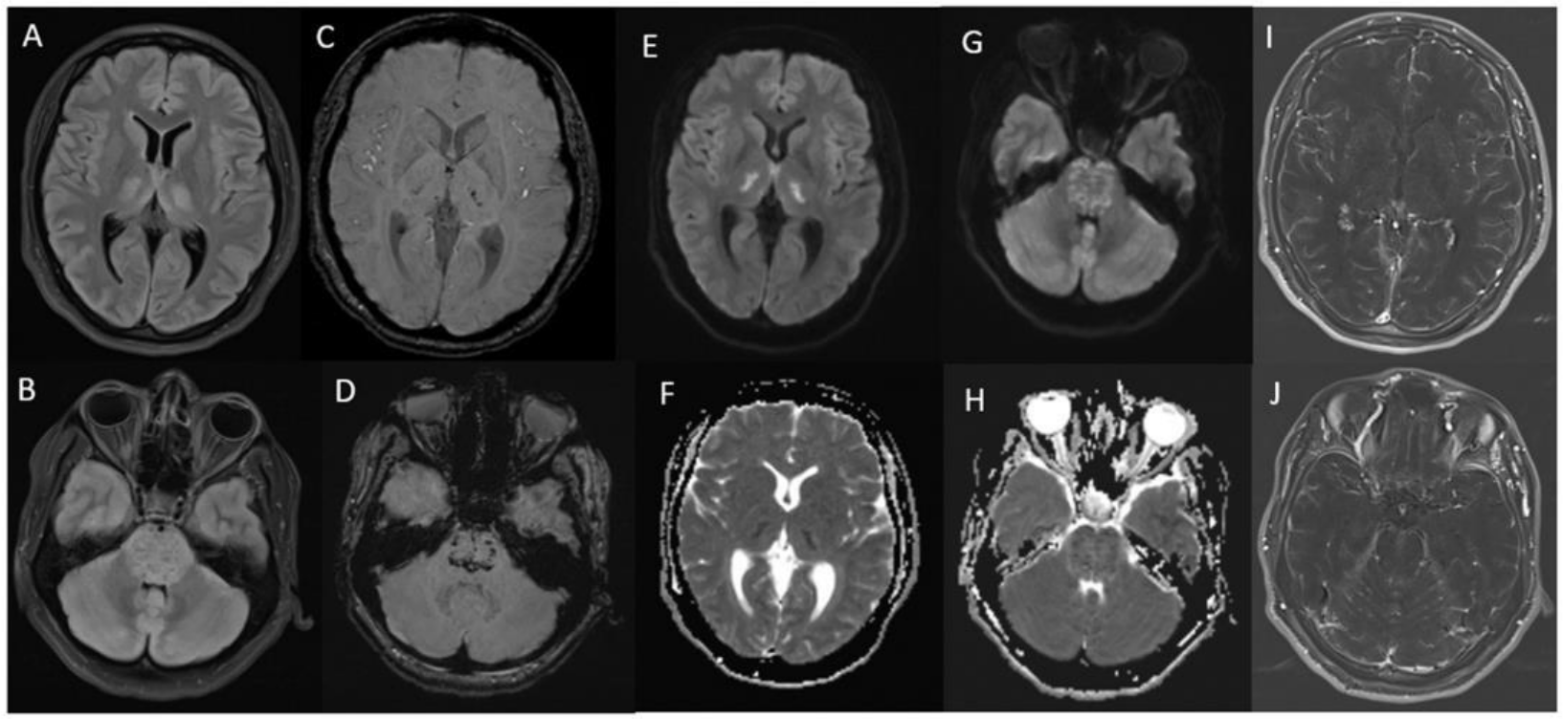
MRI brain with contrast showing acute hemorrhagic encephalomyelitis (case 2). (A,B) Axial fluid attenuated inversion recovery (FLAIR), (C,D) Susceptibility weighted imaging (SWI), (E,G) Diffusion weighted imaging (DWI), (F,H) Apparent Diffusion coefficient (ADC) sequences, (I,J) T1 post-contrast subtraction images showing bilateral almost symmetrical abnormal diffusion restriction in the thalami and swollen pons, corresponding high FLAIR signal intensity with extensive micro hemorrhages on SWI and diffuse extensive bilateral symmetrical leptomeningeal enhancement involving both cerebellar and cerebral hemispheres.
